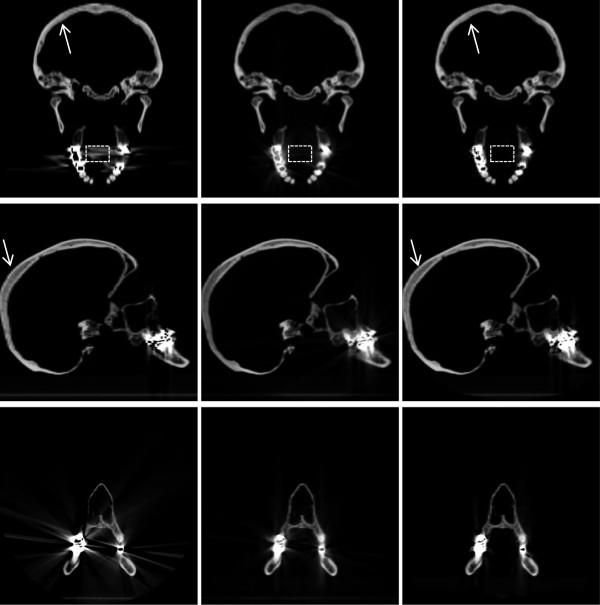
Image quality and visible detail are clearly improved. Comparison of a single CT (left) to the average (middle) and median (right) of nine tilted CTs: streaking artifacts are strongly reduced, especially in the transverse and coronal view. Details in the vicinity of the metal implants are more truthfully represented, e.g. grey values of bone tissue within the bridges and crowns. In addition, aliasing has been reduced as well, which is especially visible in the occipital bone (arrows), a beneficial side effect of averaging over multiple exposures from different angles. The bounding box shows the region of interest for the calculation of bias, error and noise centered in the oral cavity.
